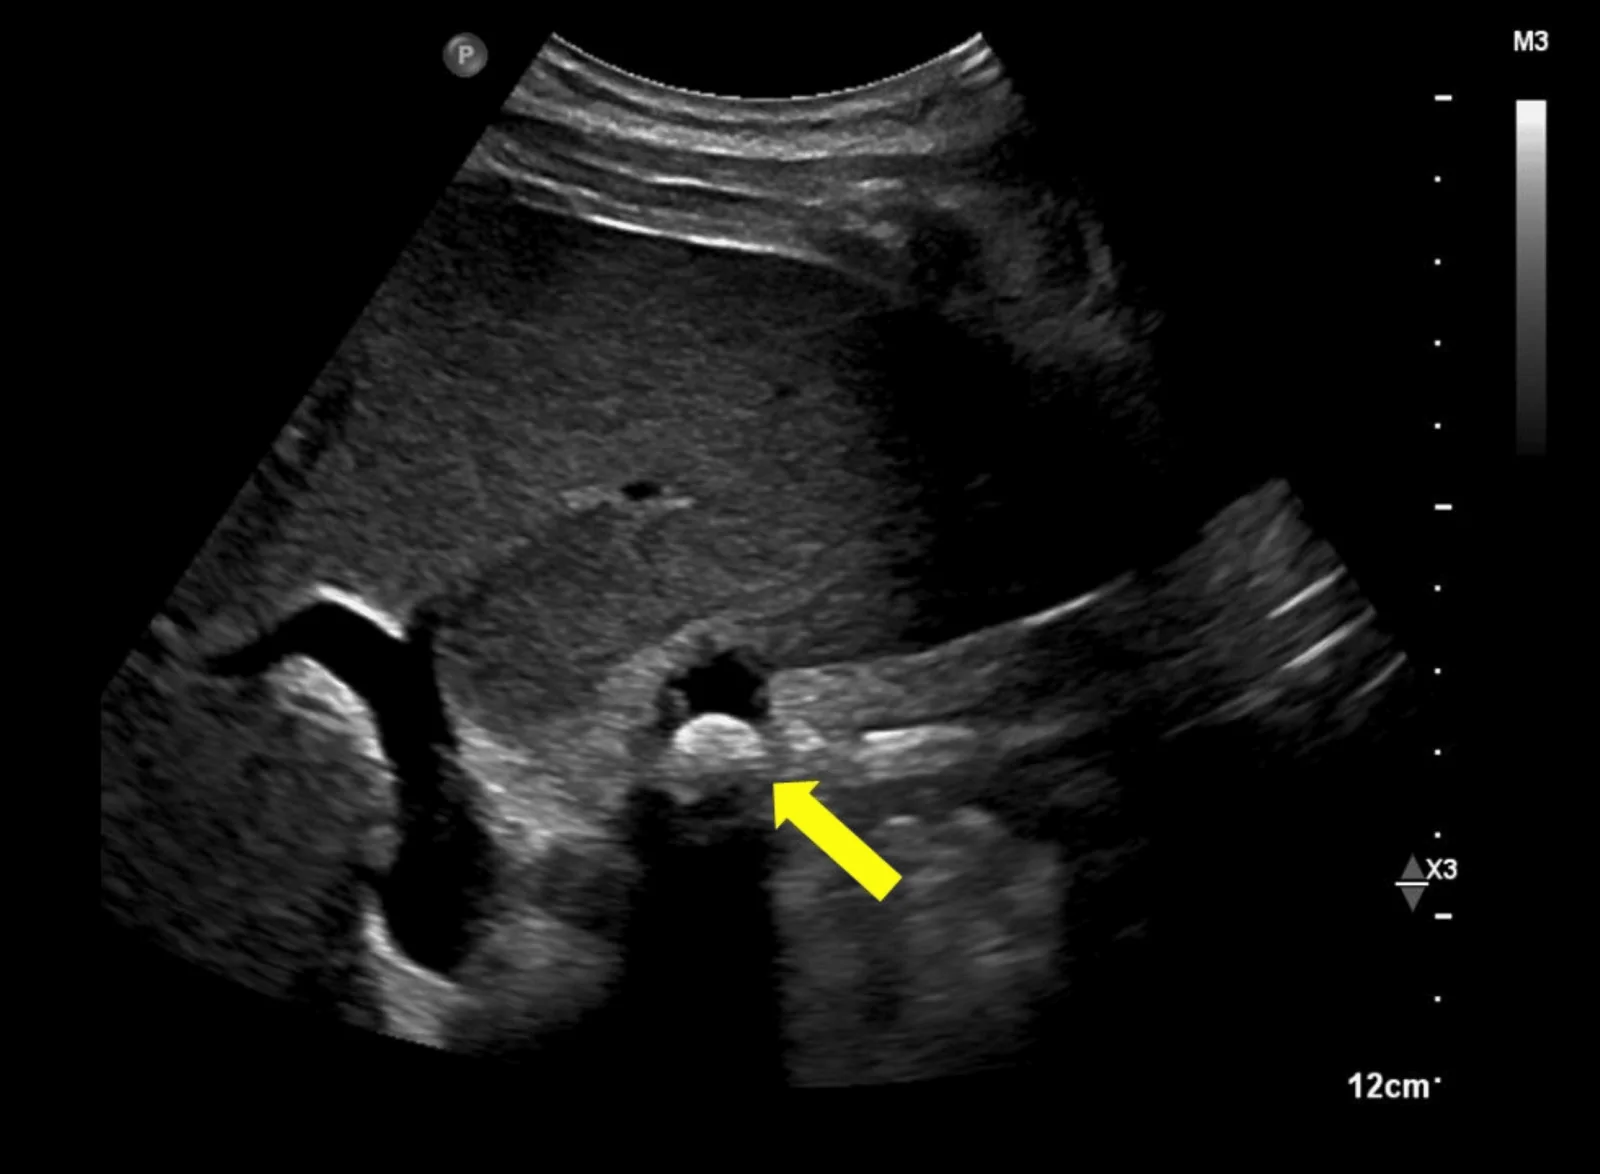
Transverse abdominal ultrasound demonstrating an echogenic gallstone (arrow) within the gallbladder, consistent with cholelithiasis.
